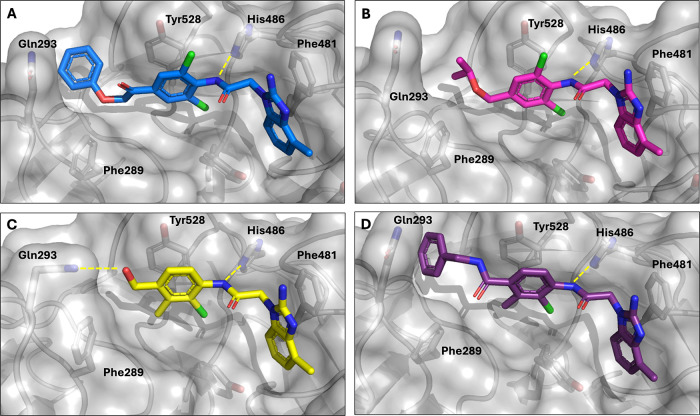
X-ray structures of cleft-targeted
